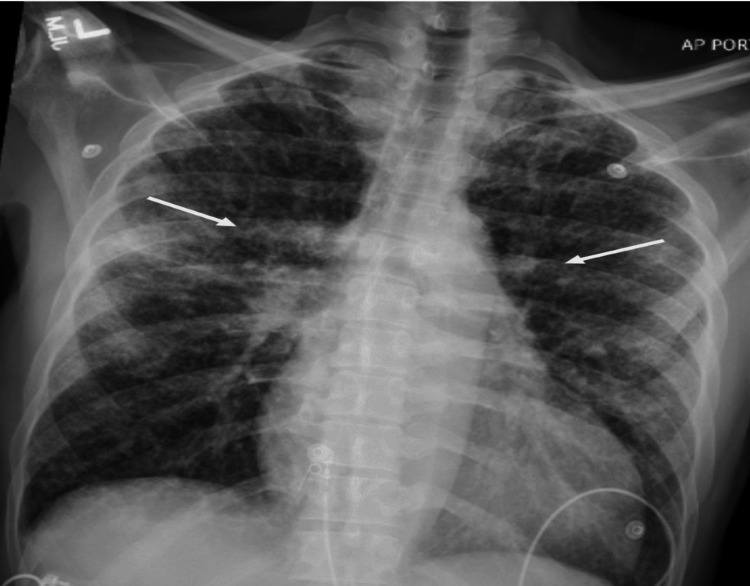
Chest X-ray (anteroposterior view) on day 10 of presentation. White arrows show worsening of bilateral hilar opacities.
